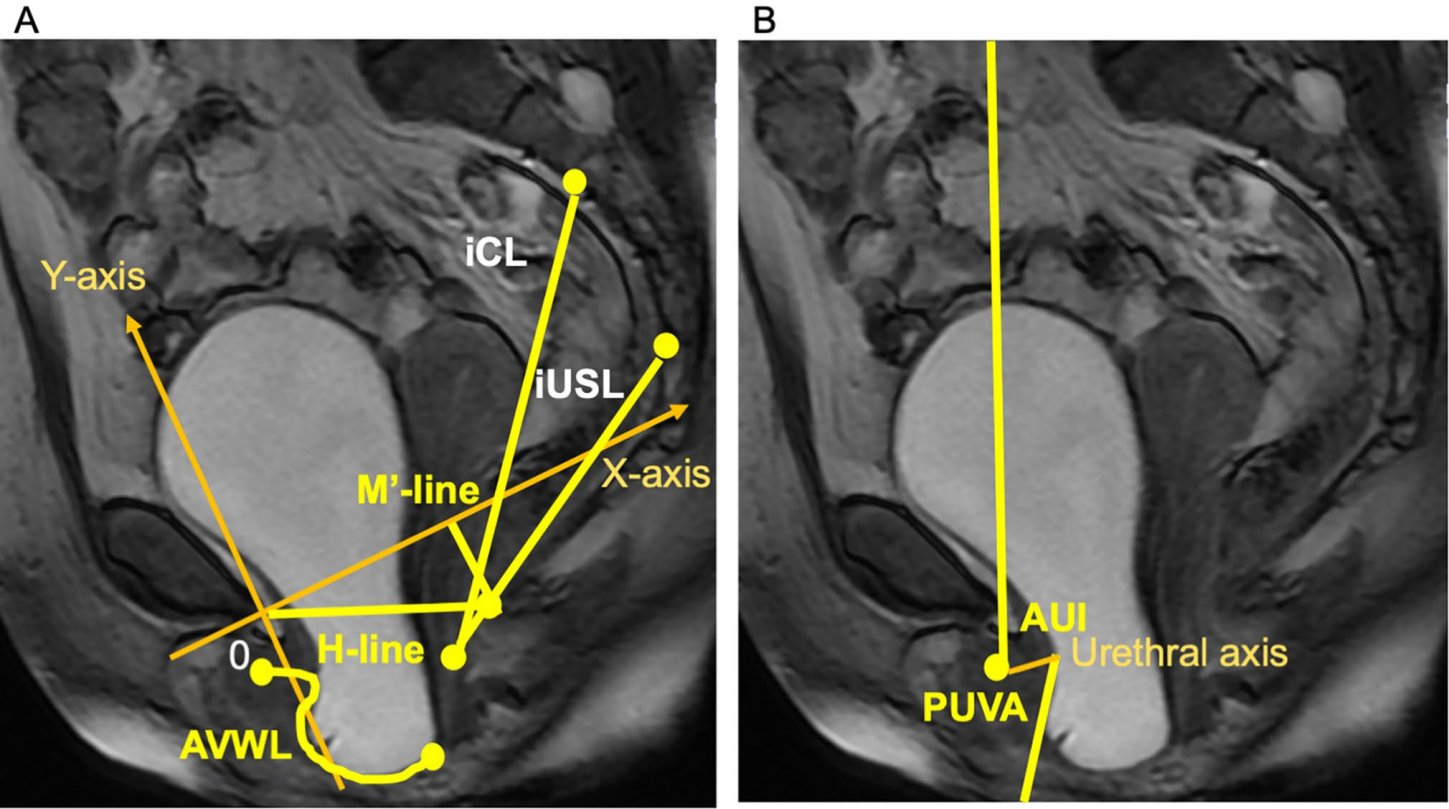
Methods to calculate parameters of pelvic organ support and bladder outlet obstruction on dynamic magnetic resonance imaging. (A) Shows measured parameters as follows: the anterior vaginal wall length (AVWL) is traced from the anterior vaginal fornix to the external urinary meatus; the length of the urogenital hiatus (H-line) is traced from origin to anorectal angle; the imaginary cardinal ligament (iCL) is traced from the anterior surface between the second and third sacral bones to the uterine cervix (C); the imaginary uterosacral ligament (iUSL) is traced from the anterior surface between the fourth and fifth sacral bones to C; the hiatal descent (M′-line) extends perpendicularly from the SCIPP line to the posterior end of the H-line. (B) Shows measured parameters for bladder outlet obstruction: the angle of urethral inclination (AUI) is the angle of the urethral axis in relation to the vertical plane; the posterior urethrovesical angle (PUVA) is the angle between the urethral axis and the posterior border of the bladder base or trigone.
